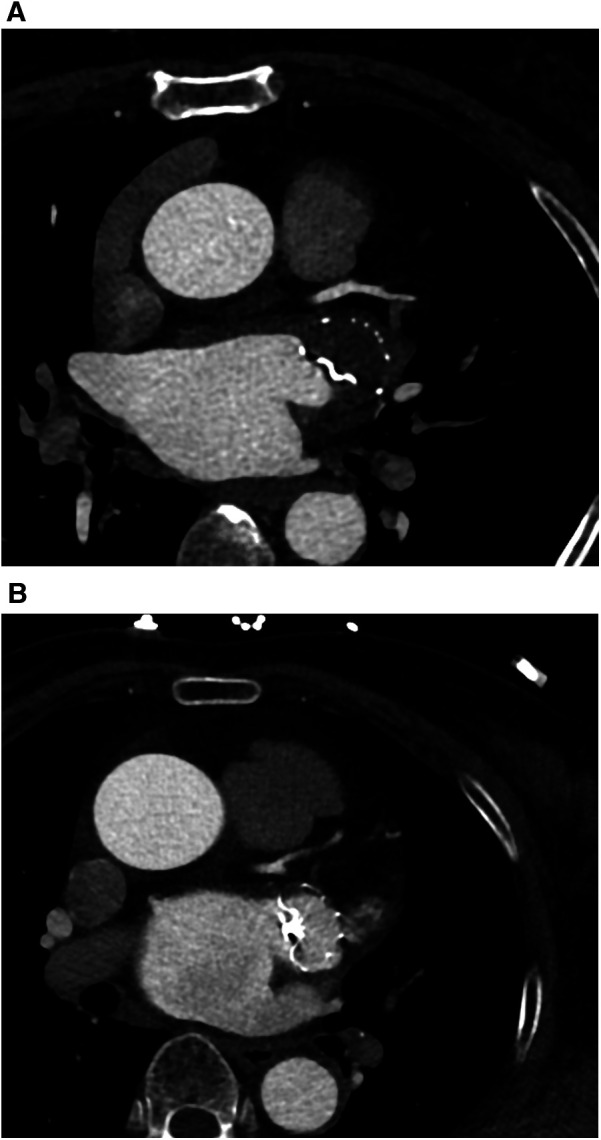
(A) One case (a 63-year-old woman with persistent atrial fibrillation) for complete occlusion at 3 months follow-up after LAAO. LAA CTA showed no residual contrast agent flow inside the LAA. (B) One case (a 68-year-old man with persistent atrial fibrillation) for incomplete occlusion at 3 months follow-up after LAAO. LAA CTA showed residual contrast agent flow inside the LAA.
